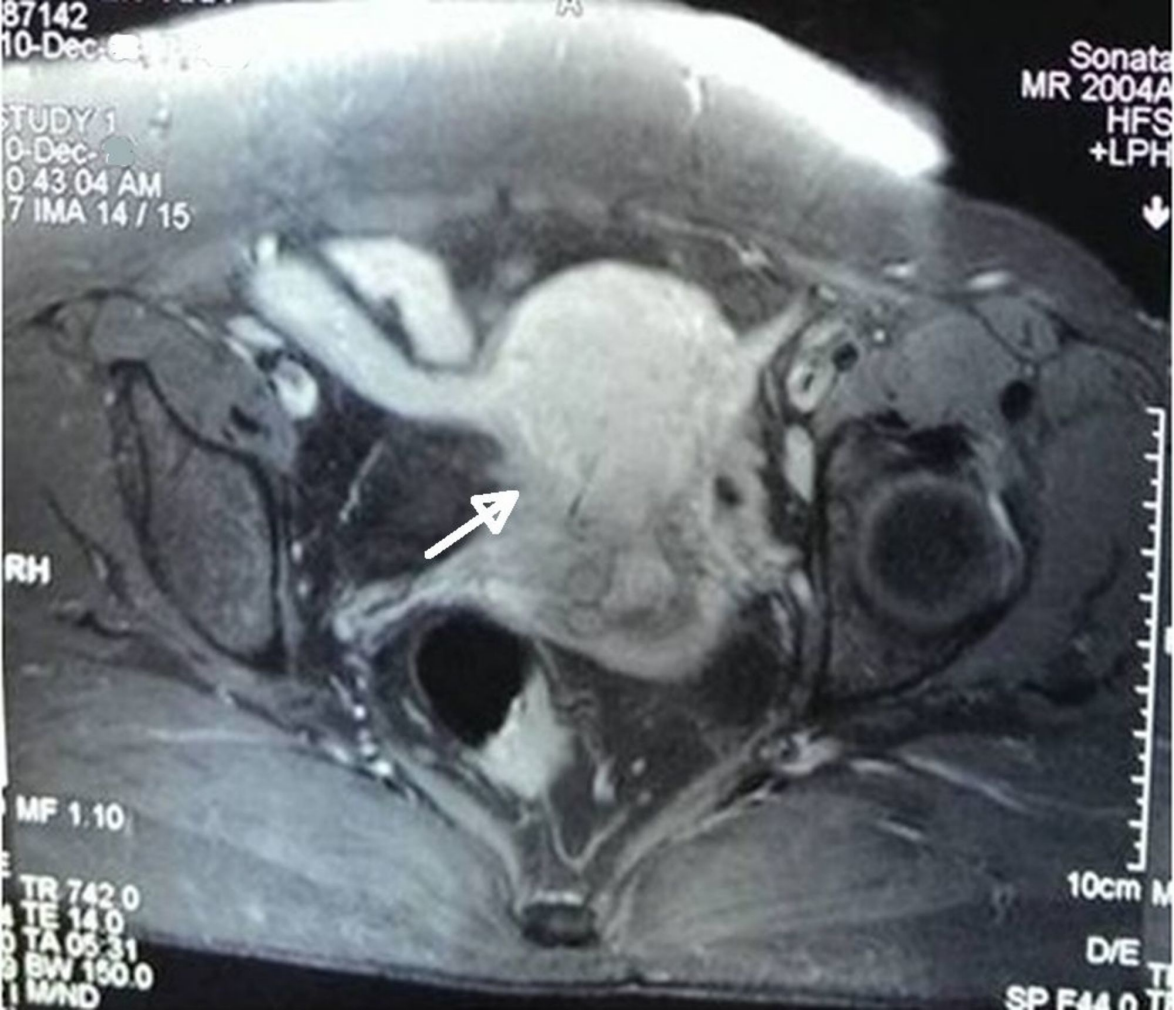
MRI image (Case 2) T2 weighted MRI image showing normally placed uterus and a fibroid polyp arising from the anterior lip of cervix (white arrow)
